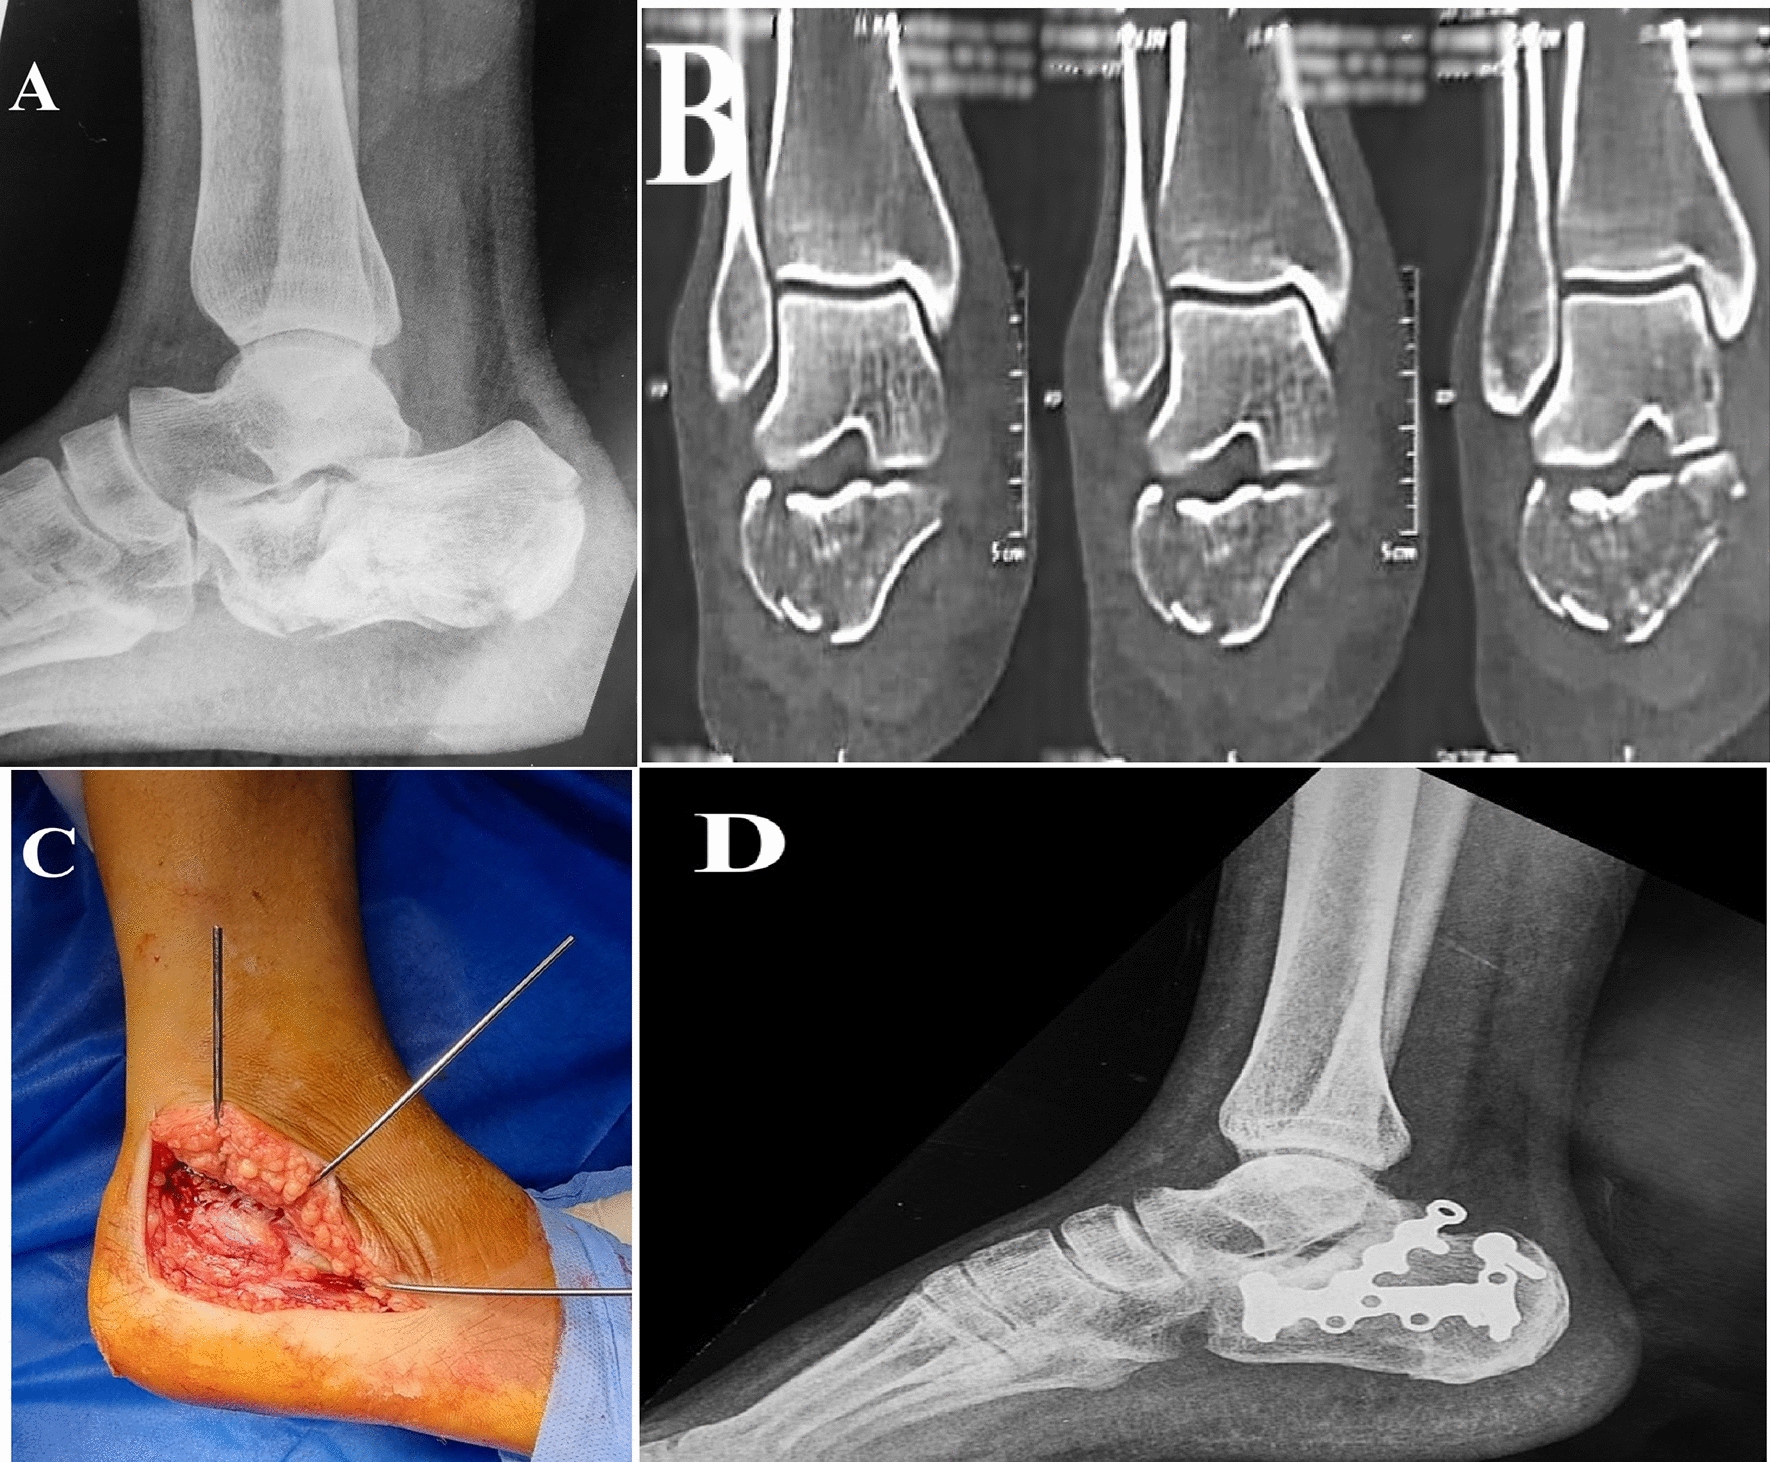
Female patient 41 years old having Sanders type III fracture treated by open reduction and internal fixation through extensible lateral approach (ELA). A Preoperative radiograph showing intraarticular calcaneal fracture with apparent joint depression. B Preoperative computed tomography showing Sanders type III fracture. C Intraoperative demonstration of the ELA. D Plain radiographs at one-year follow-up showed fracture union and maintained articular surface
